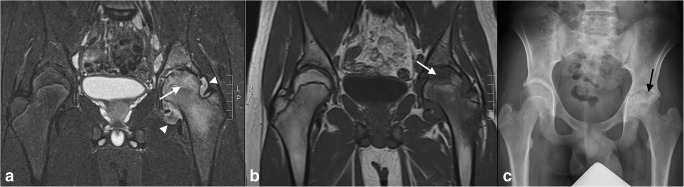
A 15-year-old boy with juvenile idiopathic arthritis and hip involvement with (a) a coronal T2-weighted fat-saturated image showing synovial thickening (arrow heads) in the left hip with extensive bone marrow oedema in the femoral head (arrow), (b) a coronal T1-weigted image showing irregular cortical linings in the scope of erosive changes (arrow) and (c) the corresponding X-ray showing joint space narrowing and cortical irregularities/erosive change in the femoral head (arrow)
